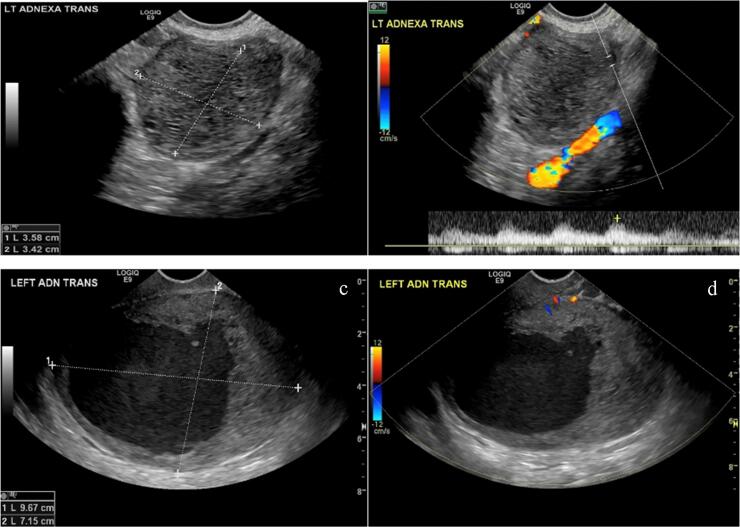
Grayscale and Doppler ultrasound images images of cystic left ovarian mass: (a and b) 12 wk 5 d exam reveals complex cyst with fairly uniform low level internal echoes and no internal blood flow, (c and d) 23 Weeks 4 day follow up exam reveals enlargement of this cystic lesion although portions are more simple appearing fluid. Note difficulty distinguishing walls of the cyst from surround ovarian stroma.
